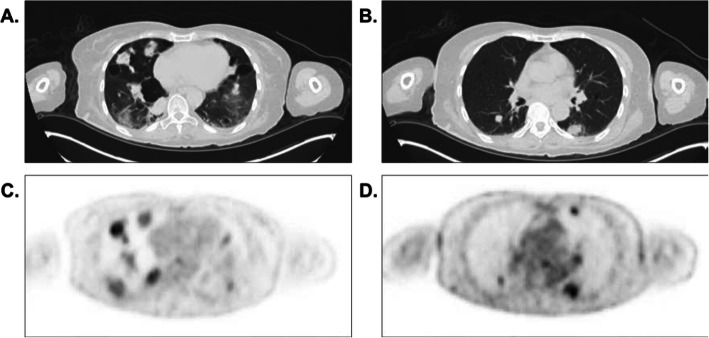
a and b. Computed tomography images with their corresponding positron emission tomography (PET) images (c and d) showing numerous pulmonary masses and nodules in the lower lung fields (a and c) and in the posterior segment of the left lower lobe (b and d)
